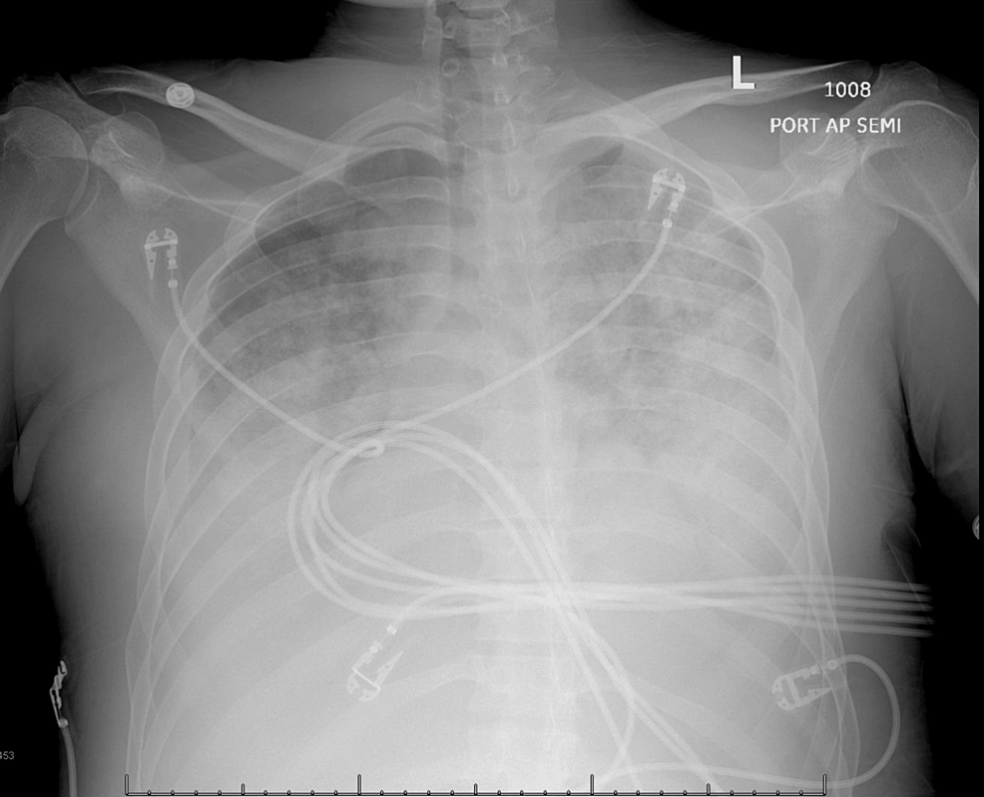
Chest X-ray shows bilateral patchy opacities with pulmonary vascular congestion.
